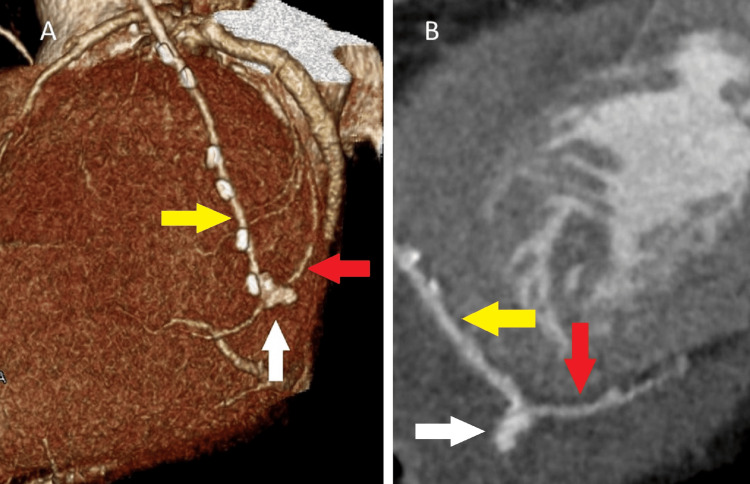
Contrast computed tomography (CT) on postoperative day five A 2.9 mm x 3.1 mm x 8.0 mm pseudoaneurysm (white arrow) was observed at the anastomosis site of the left internal mammary artery (yellow arrow) to the circumflex artery (red arrow). It was visualized only during contrast imaging, while the surrounding hematoma was not enhanced. A: Three-dimensional CT; B: Curved multi-planar reformation
